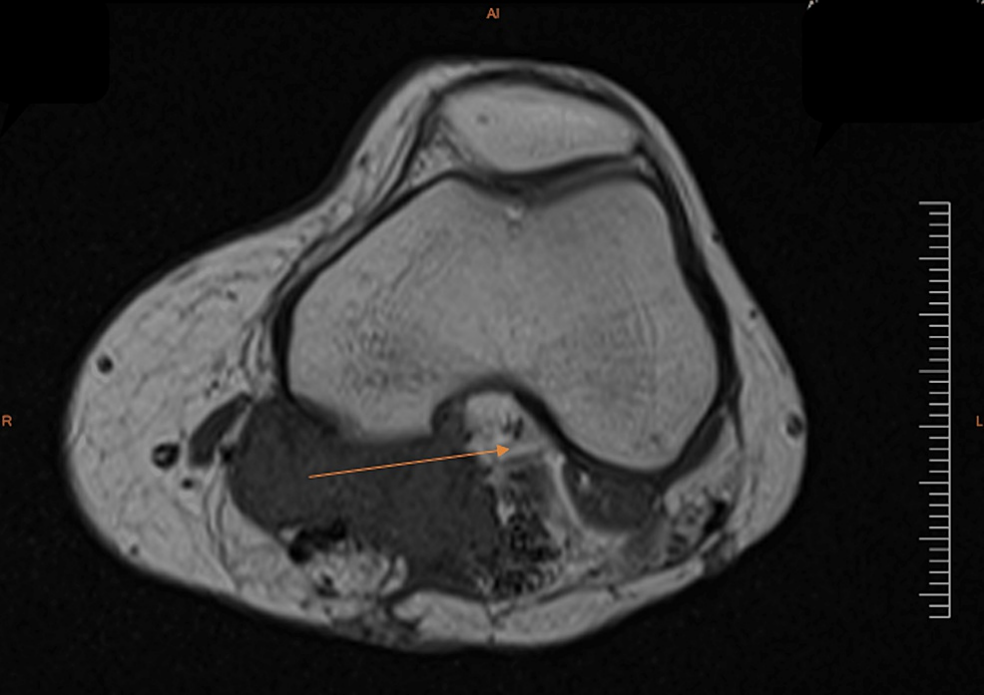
Post-operative MRI (done in December 2020) demonstrating a transverse cut through the patella and distal end of the femur of the left leg Post-surgical changes and a residual nodular mass measuring 4 x 2.5 cm were noted in the popliteal fossa. Avid enhancement was also seen along the popliteal neurovascular bundle. The arrow points to the post-operative mass that has been substantially reduced.
